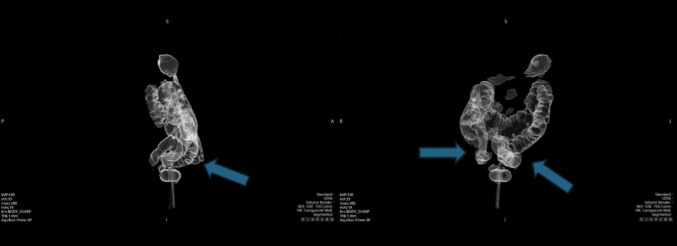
Colon-CT scan. Arrows show *“A stenosing parietal lesion is recognized at the recto-sigmoid junction. A stenosing lesion is recognized at the level of the distal descending colon, extending for a distance of approximately 2 cm. A larger stenosing lesion is recognized along the proximal third of the descending colon, extending for approximately 4 cm. No pathological lesions are recognized in the transverse colon or ascending colon”*
